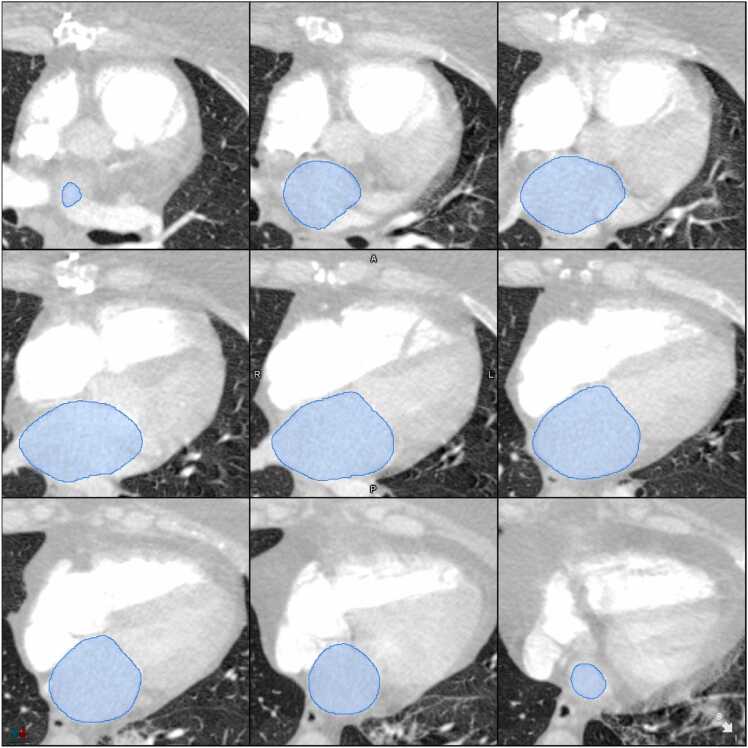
CT with segmentation. Example case: CTPA LA volume reported as normal by radiologist. CTPA AI LA volume: 125 mL. CMR AI LA volume: 106 mL. RHC wedge pressure: 29 mmHg.
